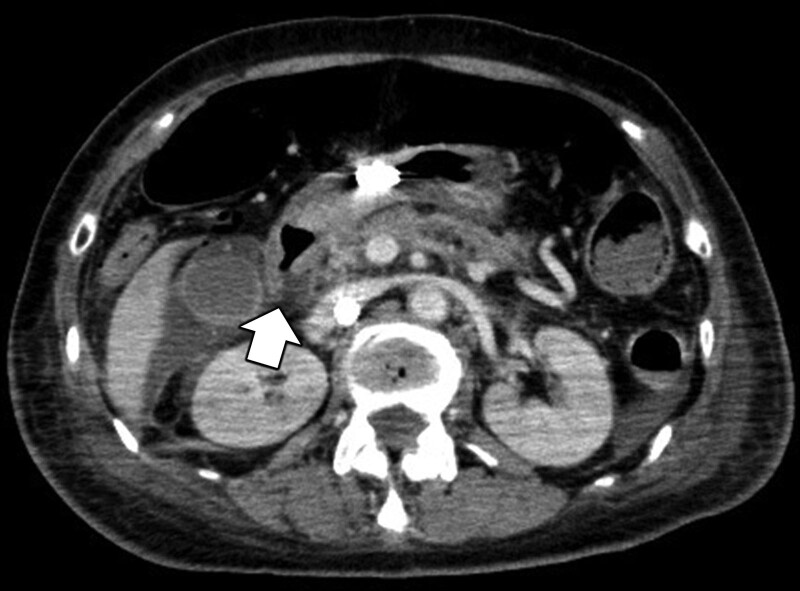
Contrast-enhanced computed tomography scan shows a poor contrast effect in the posterior wall of the duodenal bulb and perforation at this region (white arrow).
